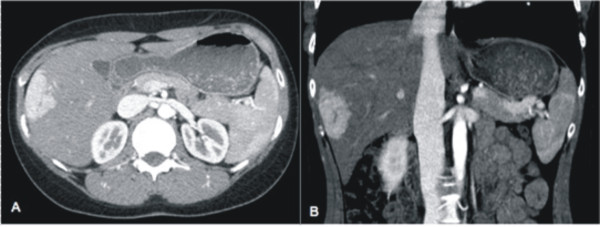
Donor candidate in whom the left lateral segments where resected for LDLT. Simultaneously, a FNH in the right lobe, incidentally detected in the CT scan, was resected.
